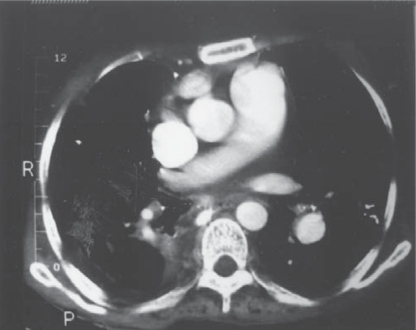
CT thorax showing right hilar lymphadenopathy
